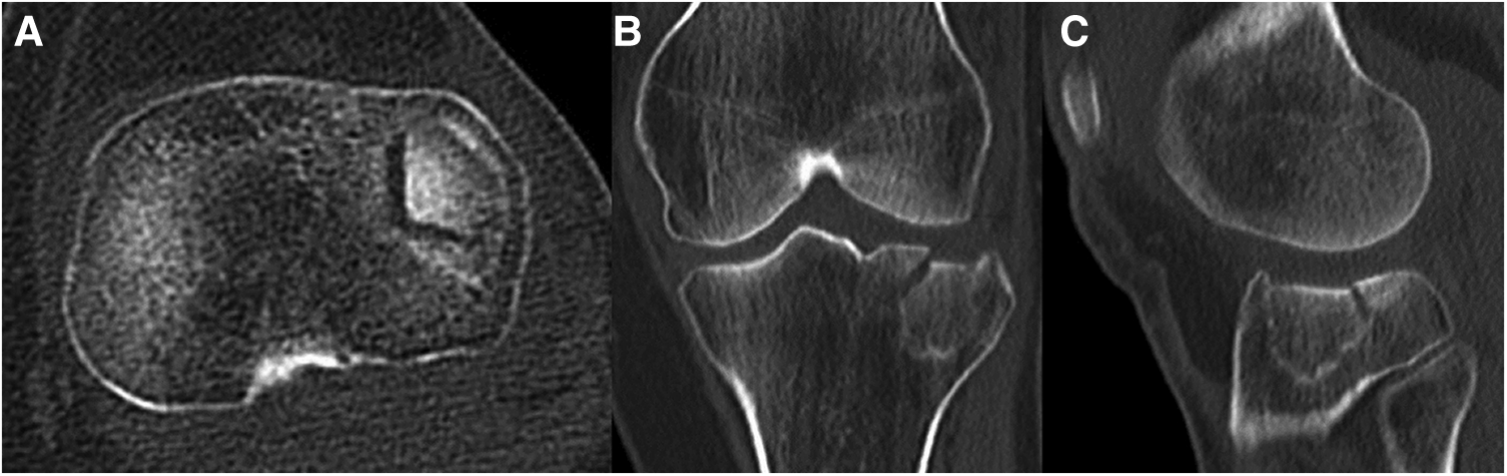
Case 2, 65year female patient, low energy knee distorsion in domestic environment. Lateral tibial plateau impression fracture (Schatzker IIIa, AO 41-B2, Zero-column, anterolaterocentral segment), as seen on the axial CT cut (A), coronal (B) and sagittal (C) reconstruction.
